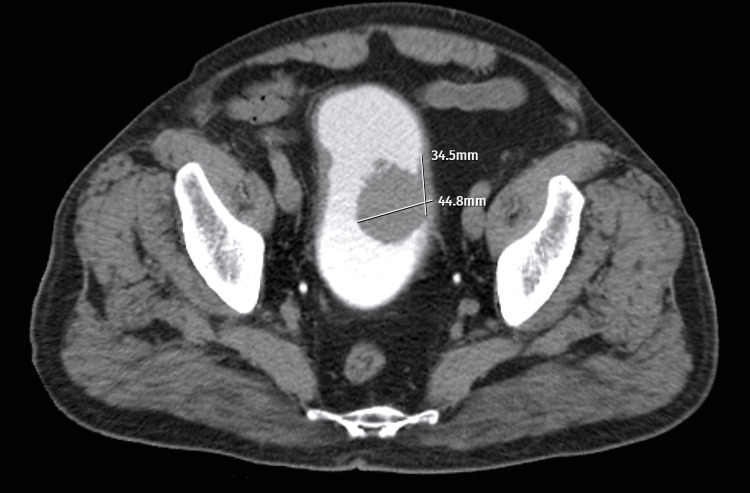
Axial CT section of the bladder tumor in a 69-year-old male patient.
